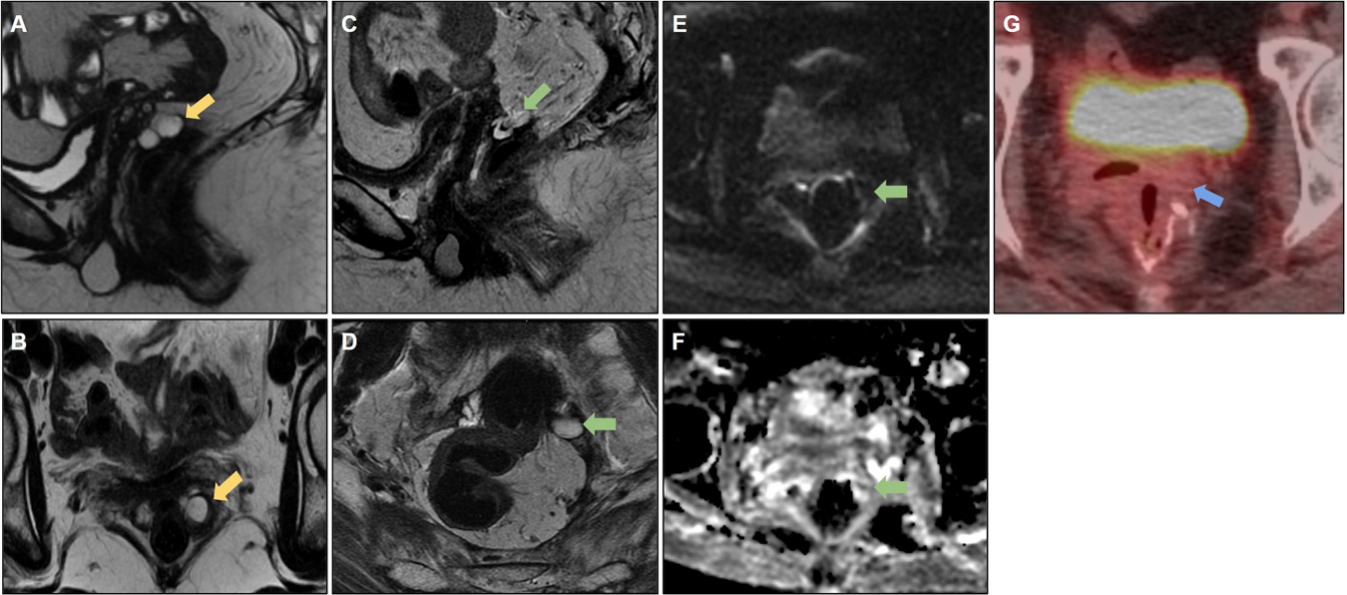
A Sagittal and B axial T2 following adjuvant chemoradiotherapy (CRT). A cystic formation was observed at the anastomosis site with peripheral intermediate T2 signal, raising suspicion for residual tissue/recurrence. The high T2 signal could potentially correspond to mucin. C, D MRI at the 6-month follow-up revealed persistent cystic formation, which remained stable with no evidence of growth. Diffusion-weighted imaging (DWI) (E) and ADC (F) do not exhibit any diffusion restrictions. G Positron emission tomography (PET)-computed tomography (CT) showing negative fluorodeoxyglucose (FDG) uptake at the location of cyst formation. Cystic formation was interpreted as a postsurgical change
